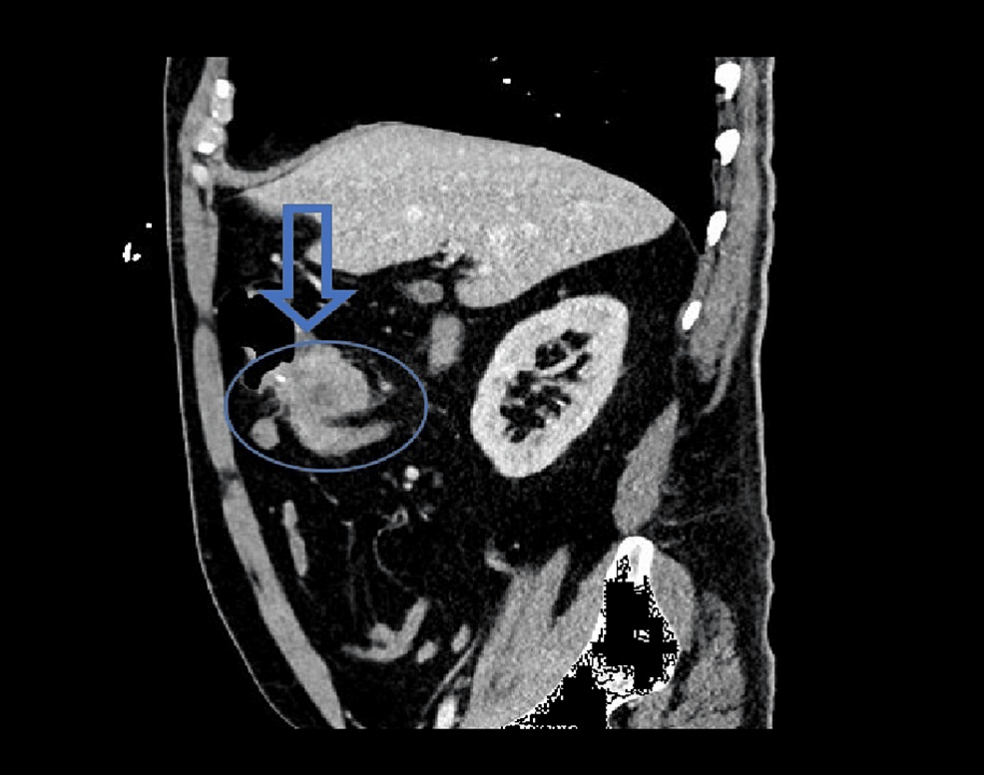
Sagittal CT scan post-contrast revealing an irregular mass at the ileocolic anastomosis site with enlarged mesenteric lymph nodes
